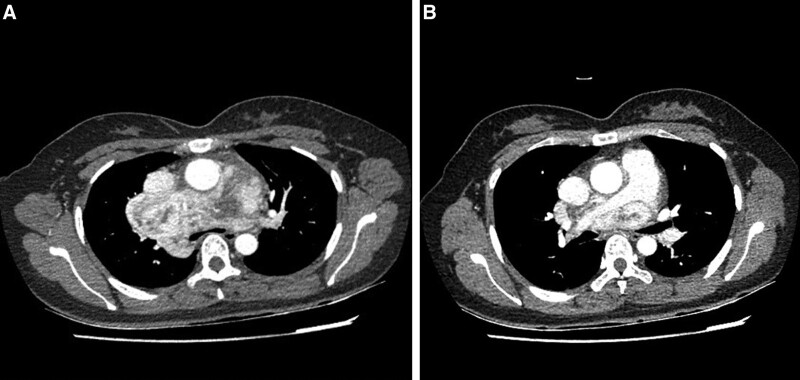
Enhanced CT of the chest indicates that the tumor is rich in blood supply. (A) The tracheal ridge is deformed by compression. (B) The tumor tissue wraps around the aorta and pulmonary artery. CT = computed tomography.
